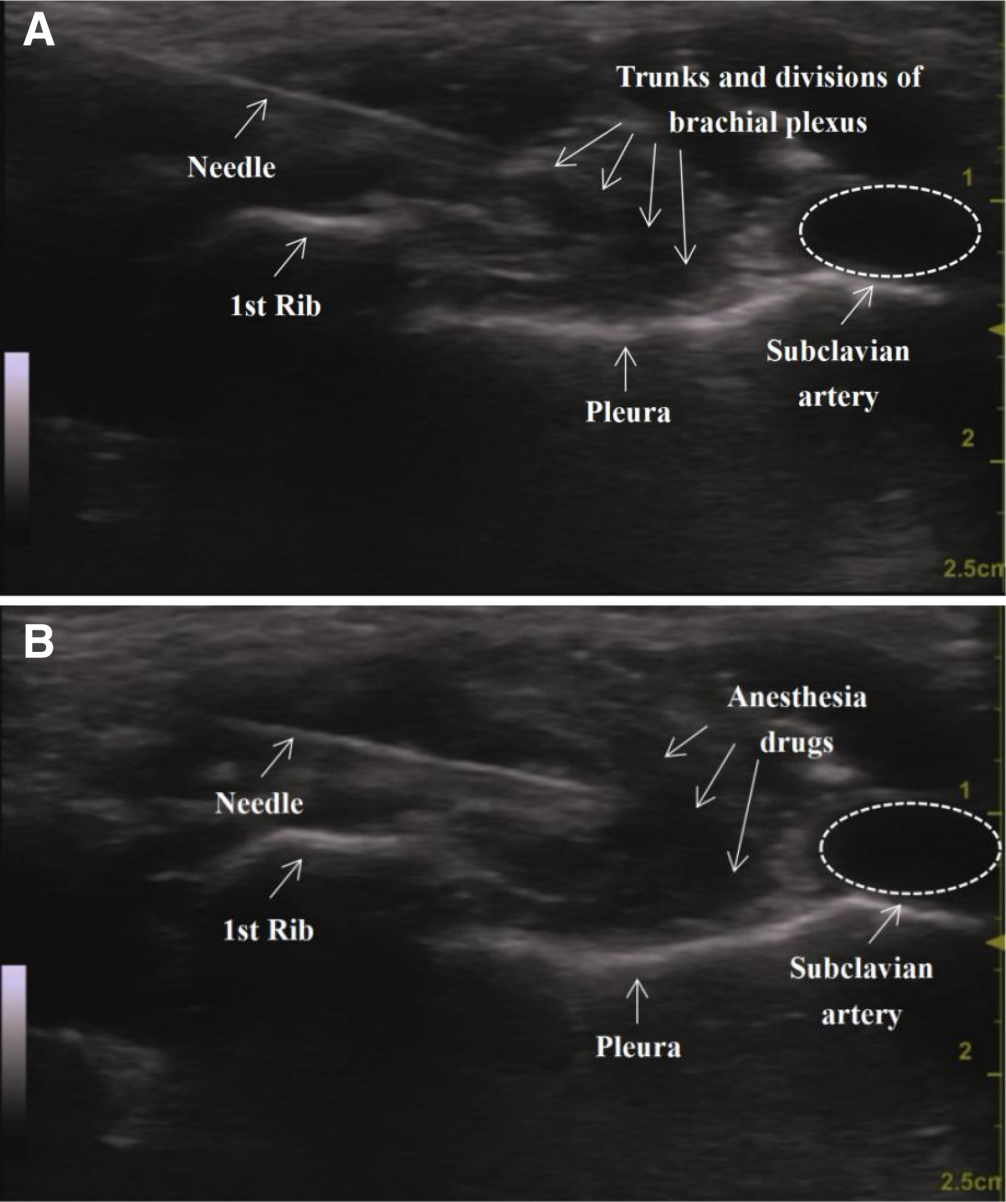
Ultrasound image of the supraclavicular approach before (A) and after (B) injection.
